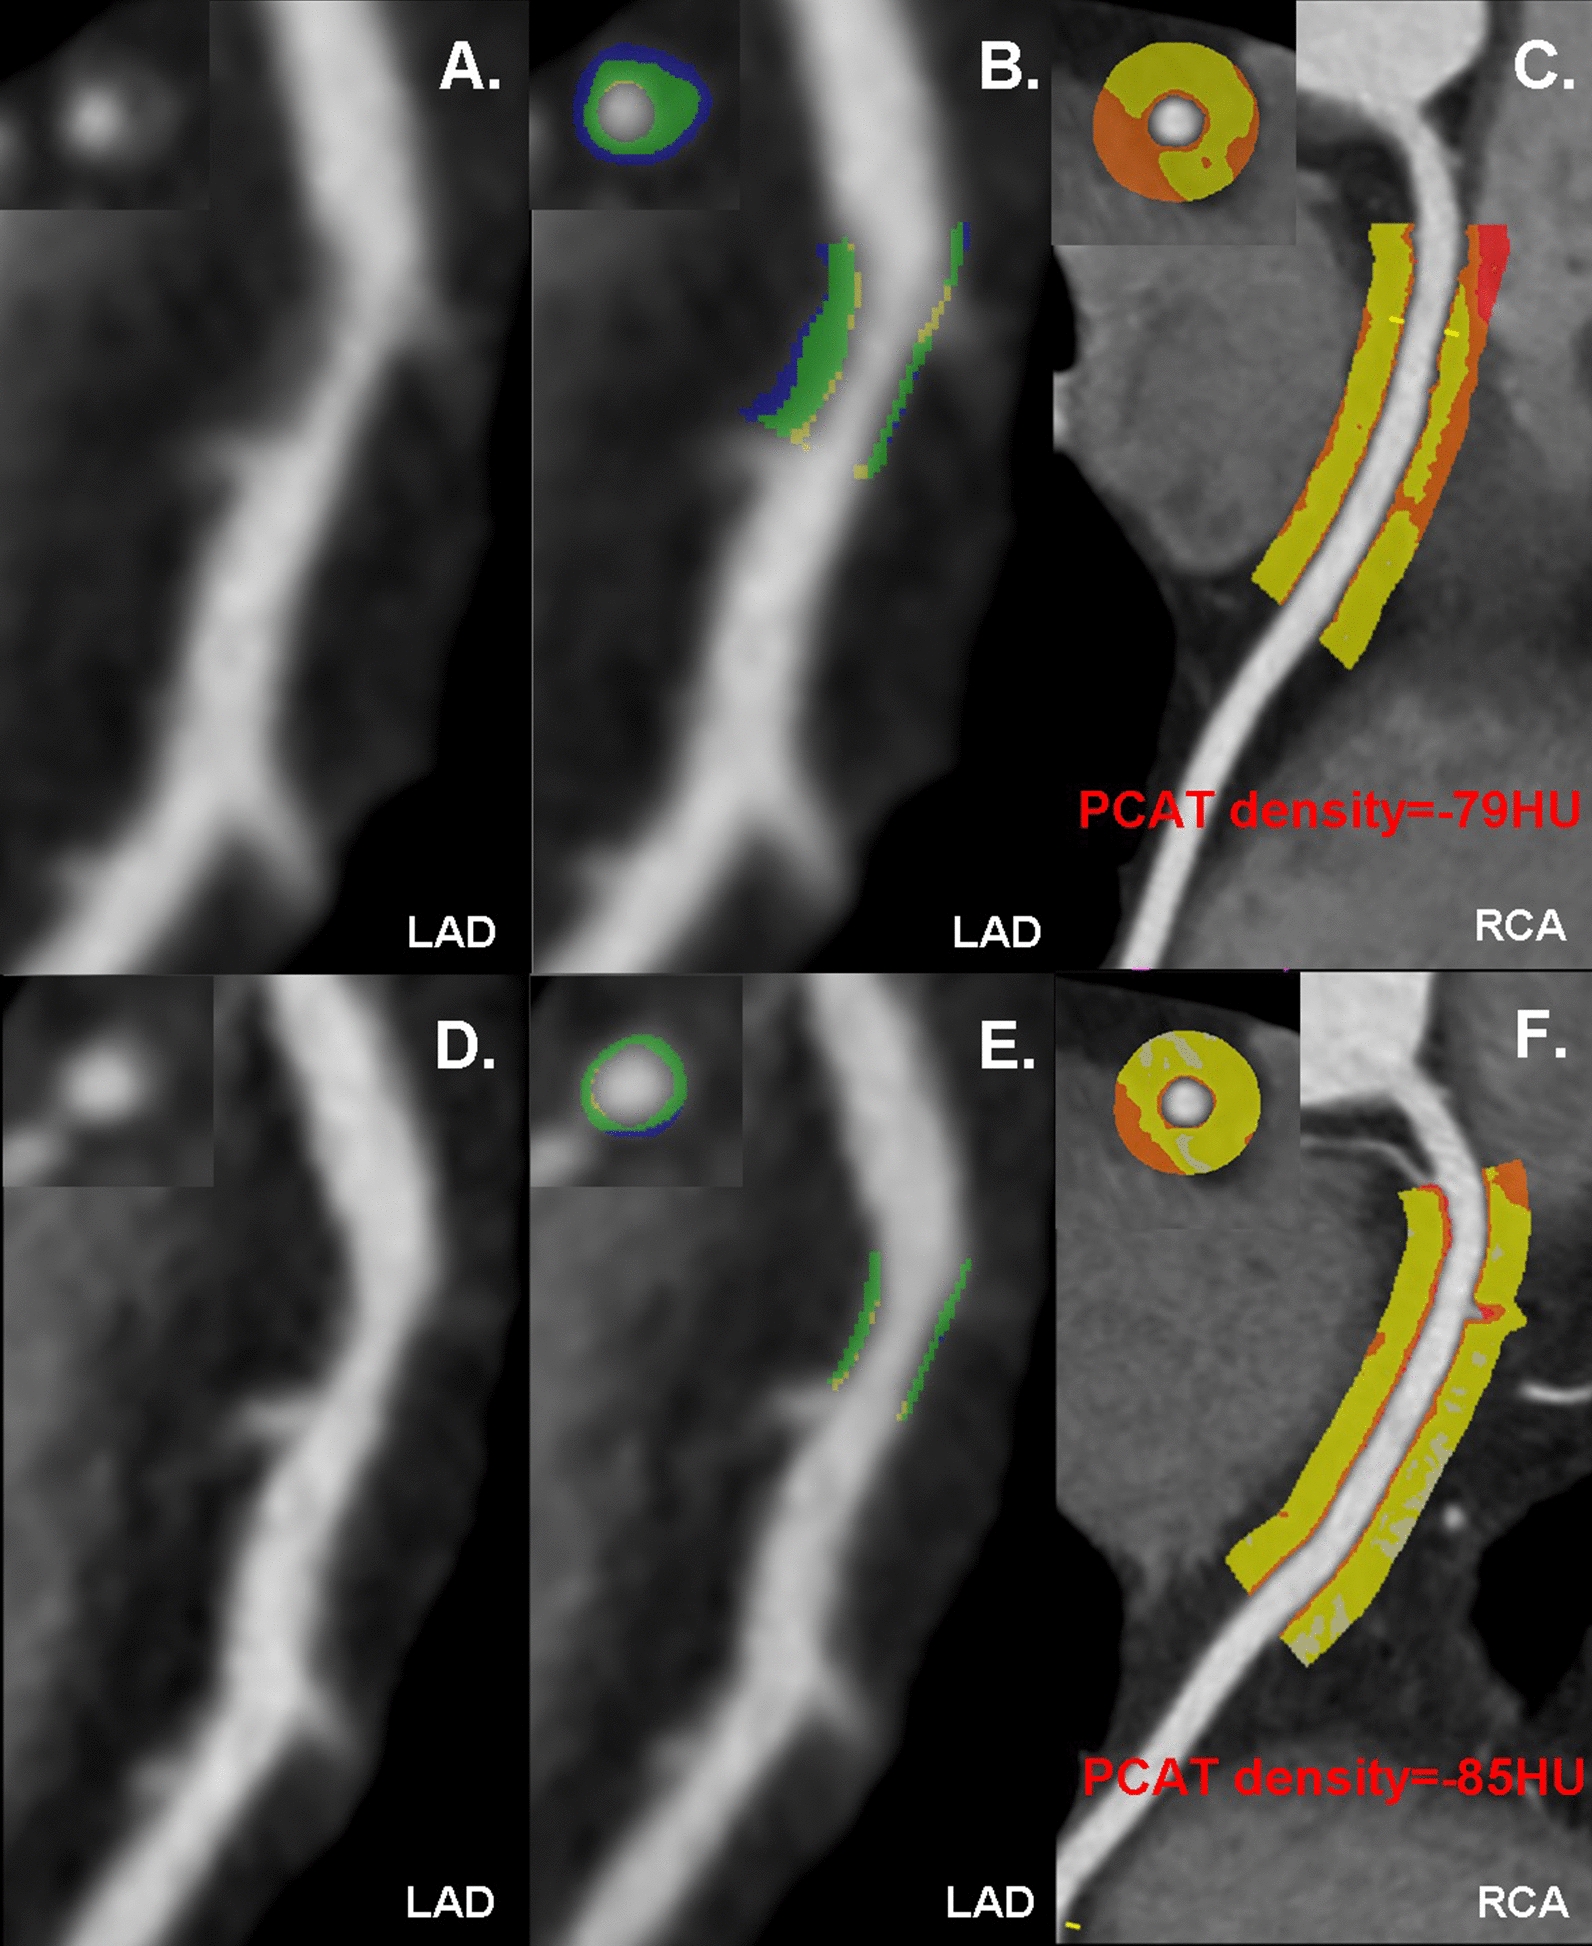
A representative case of a type 2 diabetes patients treated with evolocumab for 48-week. A–C The baseline LDL-C and Lp(a) level was 3.6 mmol/L and 64 mg/dL. Baseline CCTA revealed noncalcified plaque with moderate stenosis of middle left anterior descending artery. The total plaque volume was 132.2 mm^3^ and the PCAT density of right coronary artery was − 79HU. D–F After 48-week treatment, both the LDL-C and Lp(a) level were reduced to 1.5 mmol/L and 19 mg/dL, respectively. The follow-up CCTA revealed the lesion regression with mild stenosis. The follow-up total plaque volume decreased to 11.8 mm^3^ and PCAT density of right coronary artery attenuated to − 85HU. CCTA, coronary computed tomography angiography; HU, hounsfield unit; Lp(a), lipoprotein(a); LDL-C, low-density lipoprotein cholesterol; PCAT, pericoronary adipose tissue
